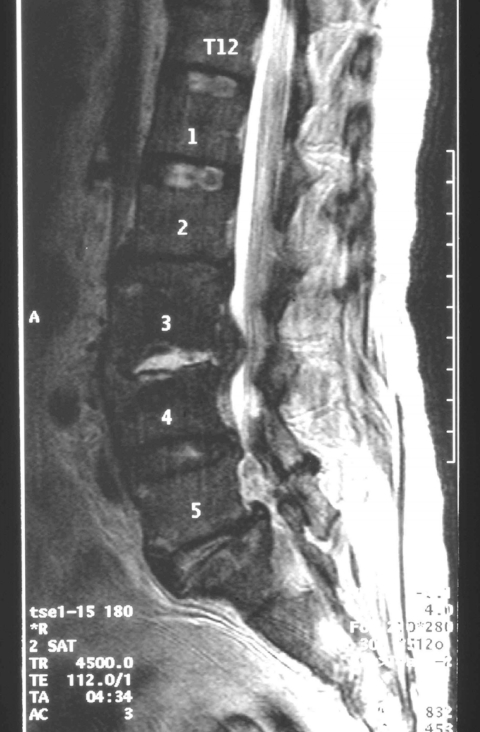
Magnetic resonance imaging scan of spine showing features compatible with discitis involving L3/L4 with surrounding paravertebral and psoas inflammation and epidural abscess.
